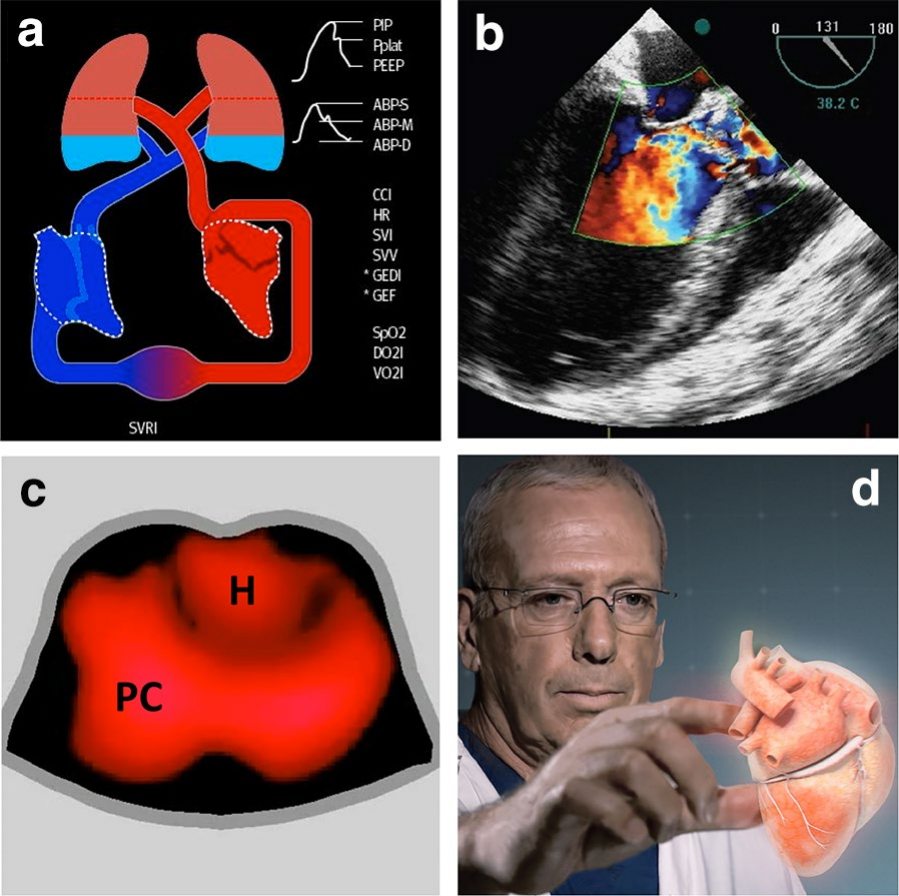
Examples of bedside digital methods useful to “look” at the cardiopulmonary circulation. a Graphical display, HemoSight screen from Mindray with permission; b echocardiography Doppler, c electrical impedance tomography, *H* heart, *PC* pulmonary circulation, from Dixtal with permission; d digital holography, downloaded from Realviewimaging.com
